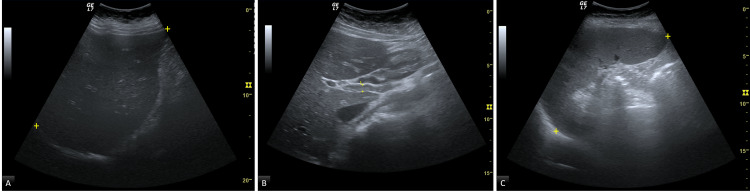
Abdominal ultrasonography A: Enlarged liver, 191mm, without focal lesions. B: Common biliary duct dilatation, 6mm, without cholelithiasis. C: Splenomegaly, 154mm. Structures marked between the yellow crosses.
